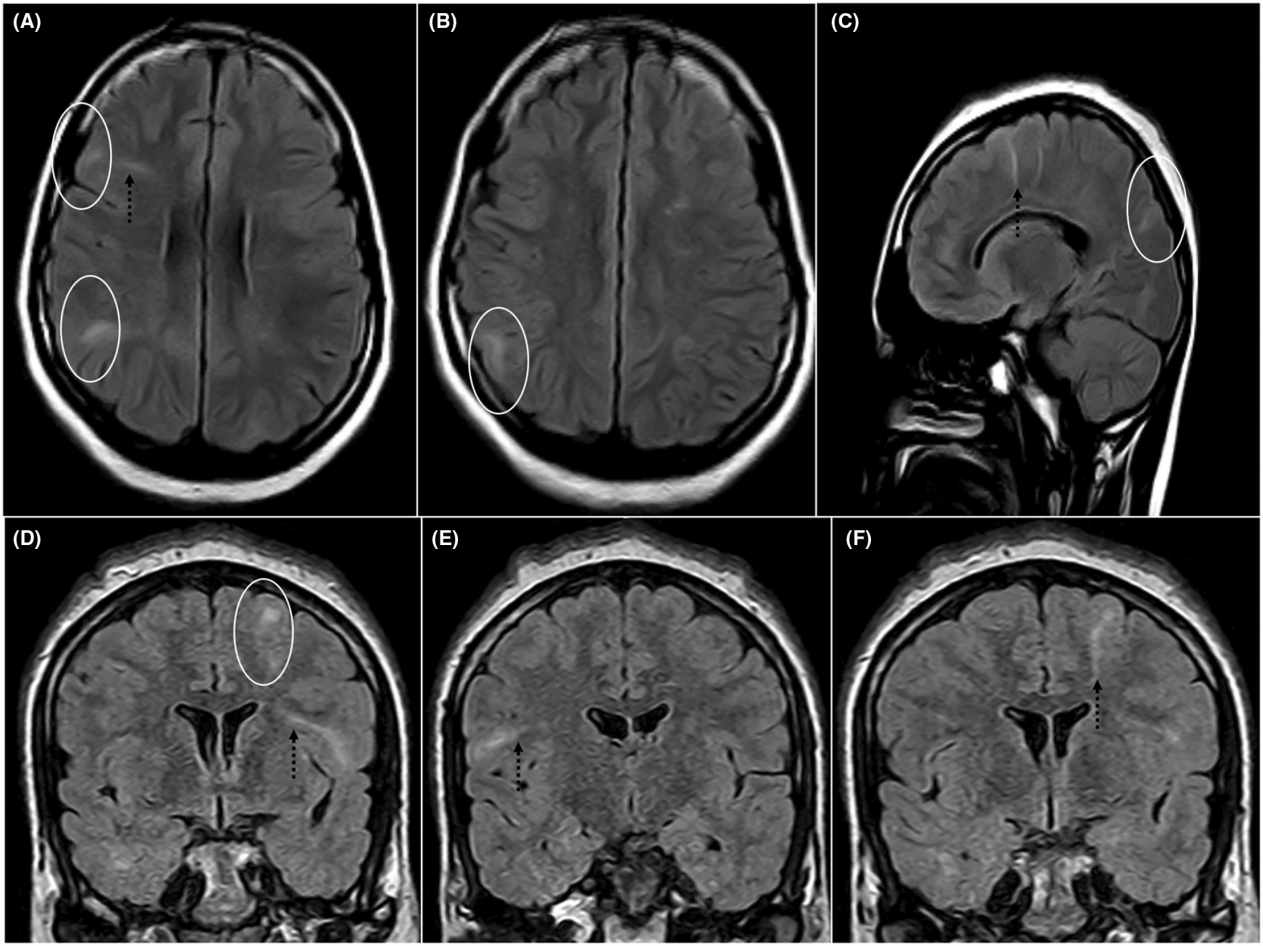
Axial (A, B), sagittal (C), and coronal (D, E, F) FLAIR images showing cortical and subcortical areas of FLAIR hyperintensities (white circles) suggesting tubers and linear hyperintensities extending from cortex suggesting radial bands.
